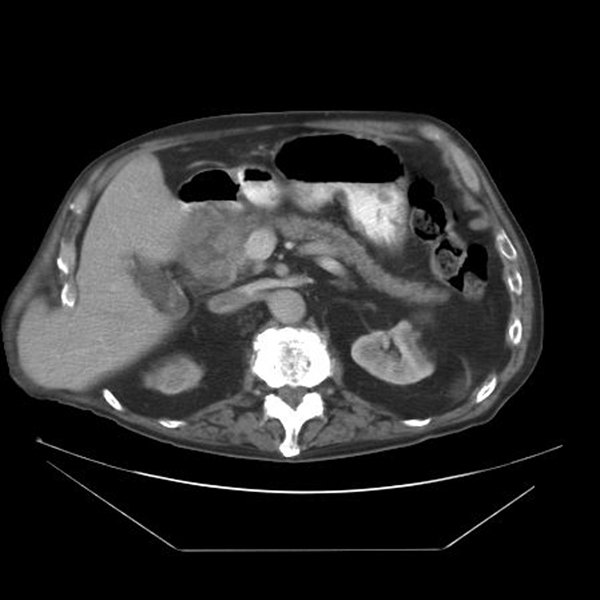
Computed tomography scan of the abdomen showing a mixed attenuation mass of the head of pancreas.
